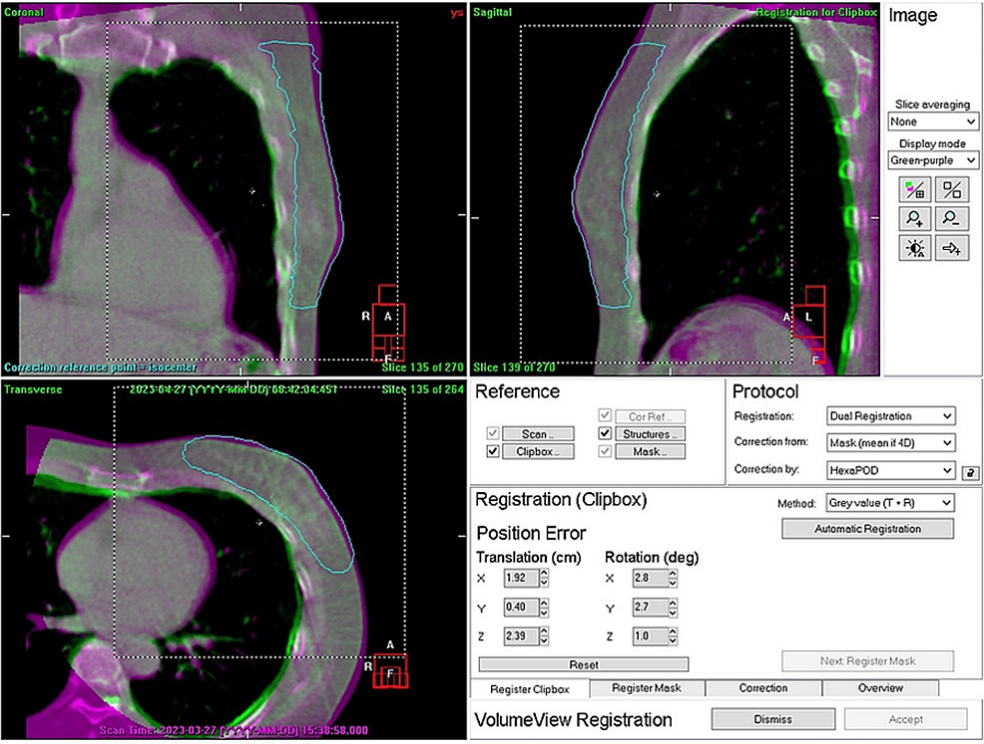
First calculation step of the chest wall to the heart distance relative to the plan. Before the treatment, a half-arc cone-beam CT (CBCT) image with a 200° gantry rotation was acquired during four repeated deep inspiration breath-holds, each spanning 50° with a 10-second breath-hold followed by five-second free breathing. Chest wall registration between the planning CT and the CBCT images was conducted using gray value matching inside a dotted white clip box. Subsequently, a manual adjustment was made as needed to match the inner chest walls, ensuring no extra doses to healthy lung tissues. The coordinate system of the Elekta linac is based on the IEC 61217 fixed coordinate system except for rotation.
